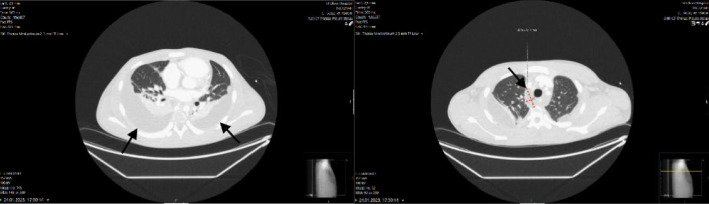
CT showed increased pleural effusions and fluid in the mediastinum.
